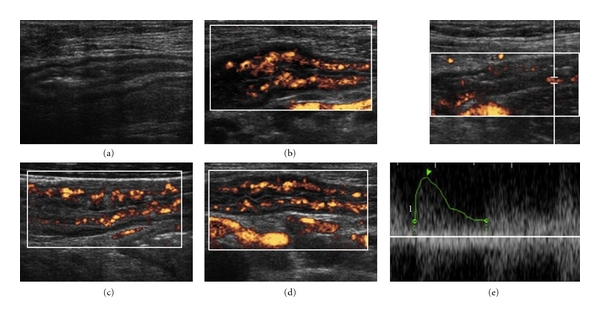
PDs. Wall thickening in B mode (a), in PD (b), (c), (d), and arterial doppler spectrum (e).
